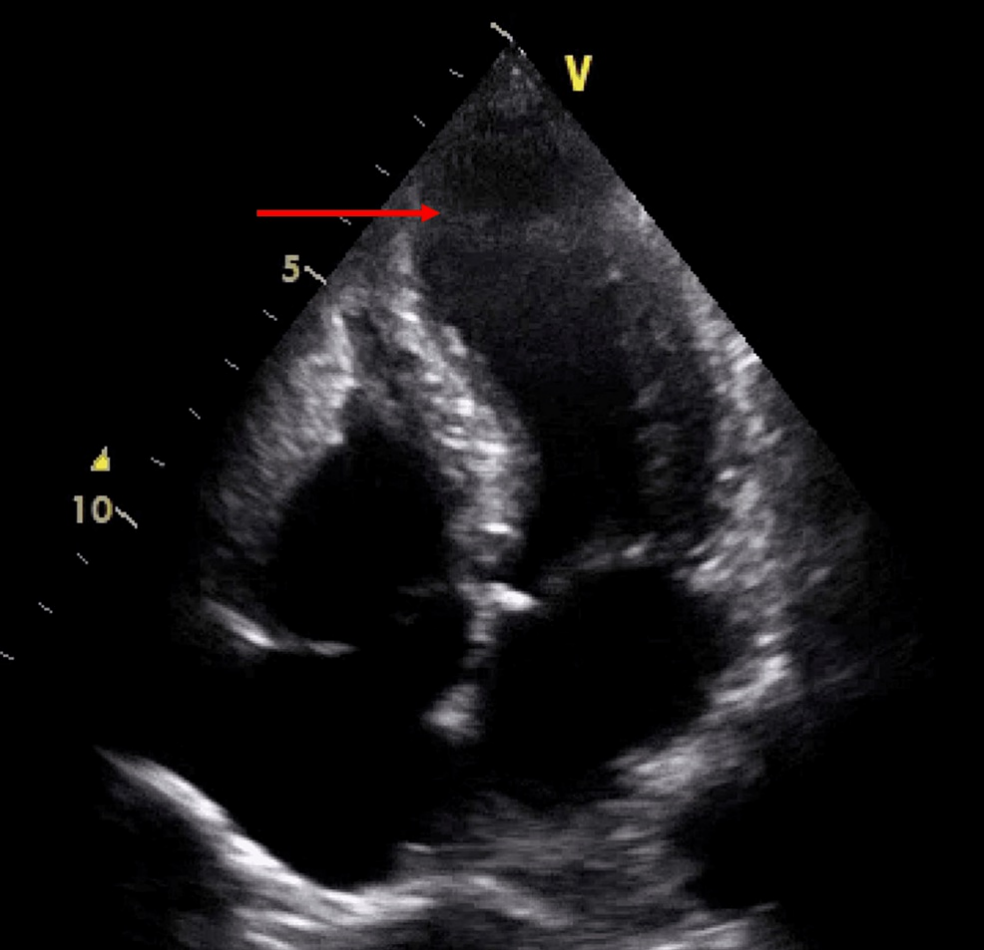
Apical four-chamber view of echocardiography showing apical hypokinesia and ballooning during ventricular systole
